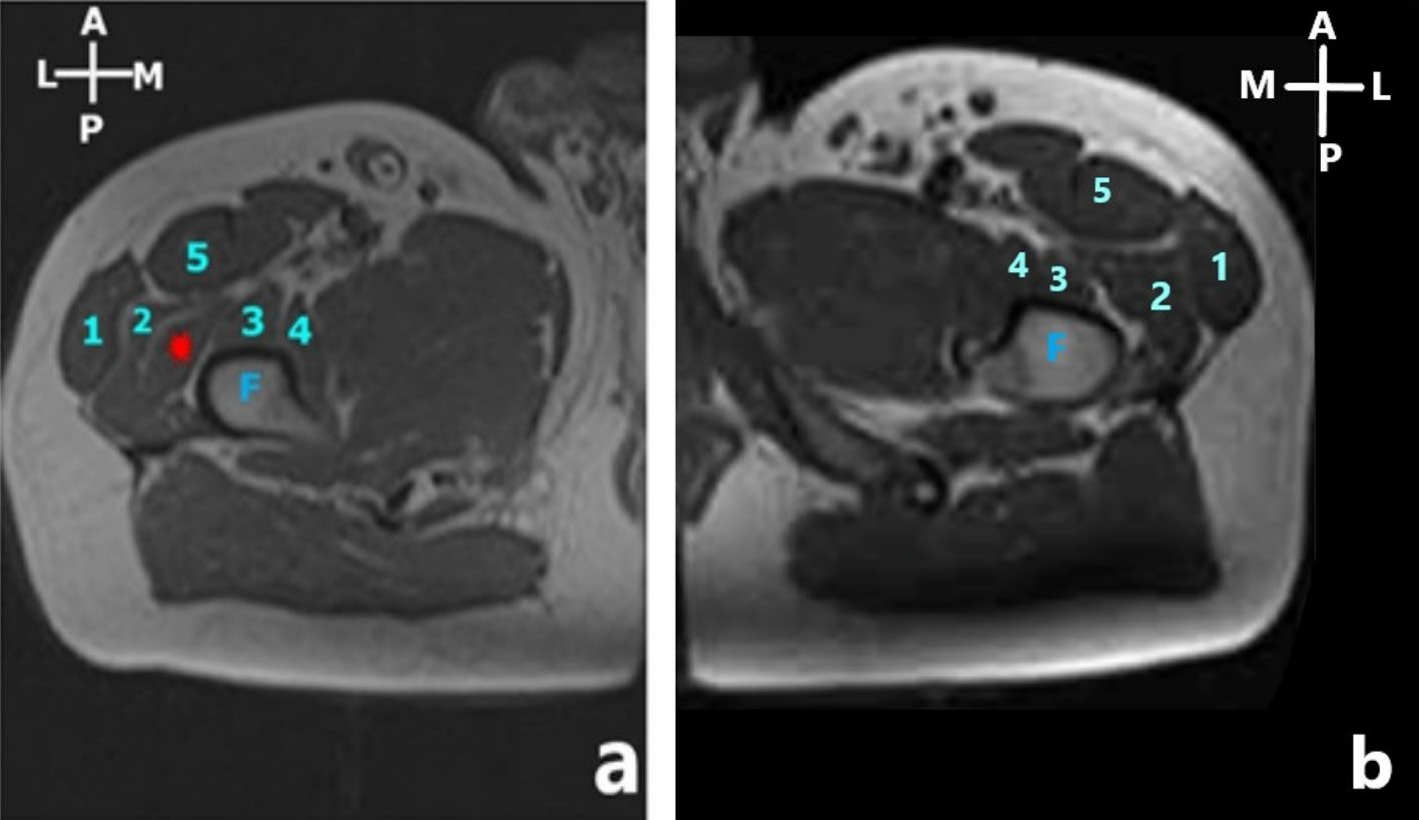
T1 weighted axial MR imaging of the lower limb of a patient; 1—tensor fascia lata, 2—vastus lateralis, 3—vastus intermedius, 4—vastus medialis, 5—rectus femoris, F—femur. The additional muscle head () is depicted in between the vastus lateralis and vastus intermedius (a) and absence of additional muscle head is depicted in (b).
